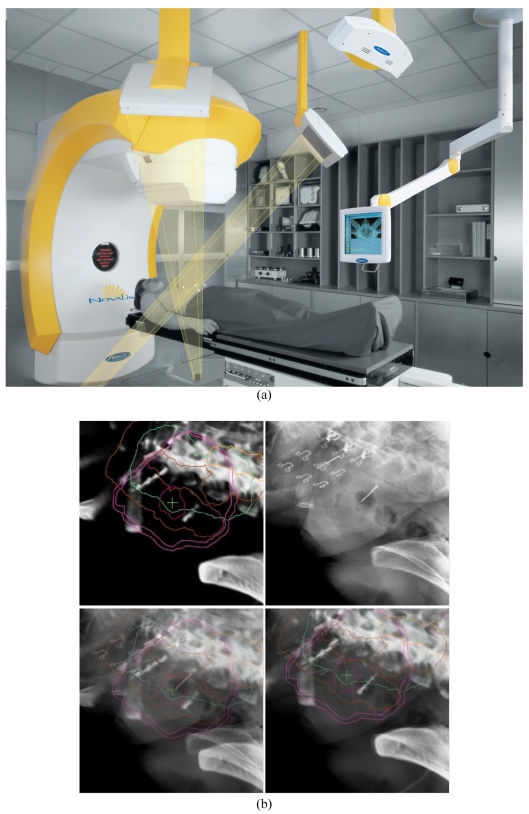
(a) The Brainlab Novalis stereotactic linear accelerator includes two orthogonal diagnostic x-ray tubes and flat panel imagers to provide image-guided 3D patient alignment. (b) Example of Novalis image alignment. Upper left panel is DRR showing expected image (from one of the two imagers). Upper right panel shows actual X-ray. Lower left panel shows overlay of the DRR and X-ray image. This information from the two orthogonal X-ray systems plus full CT data set for computation of DRRs allows calculation of patient shift to produce correct alignment. The post-shift image overlay is shown in the bottom right panel. Note the quality of the image alignment both by the bony landmarks and the implanted fiducial markers.
